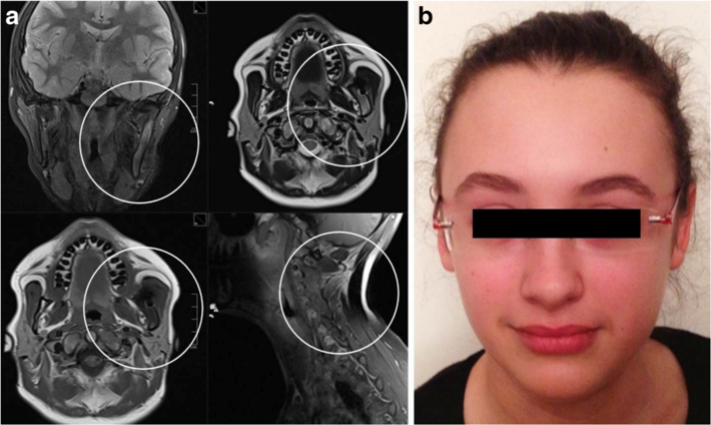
MRI (a) and the patient (b) 6 months after therapy. The MRI shows a distinct decrease of the thickening of the left mandible and the soft tissue mass in the posterior aspect of the cervical spine (*circles*)
